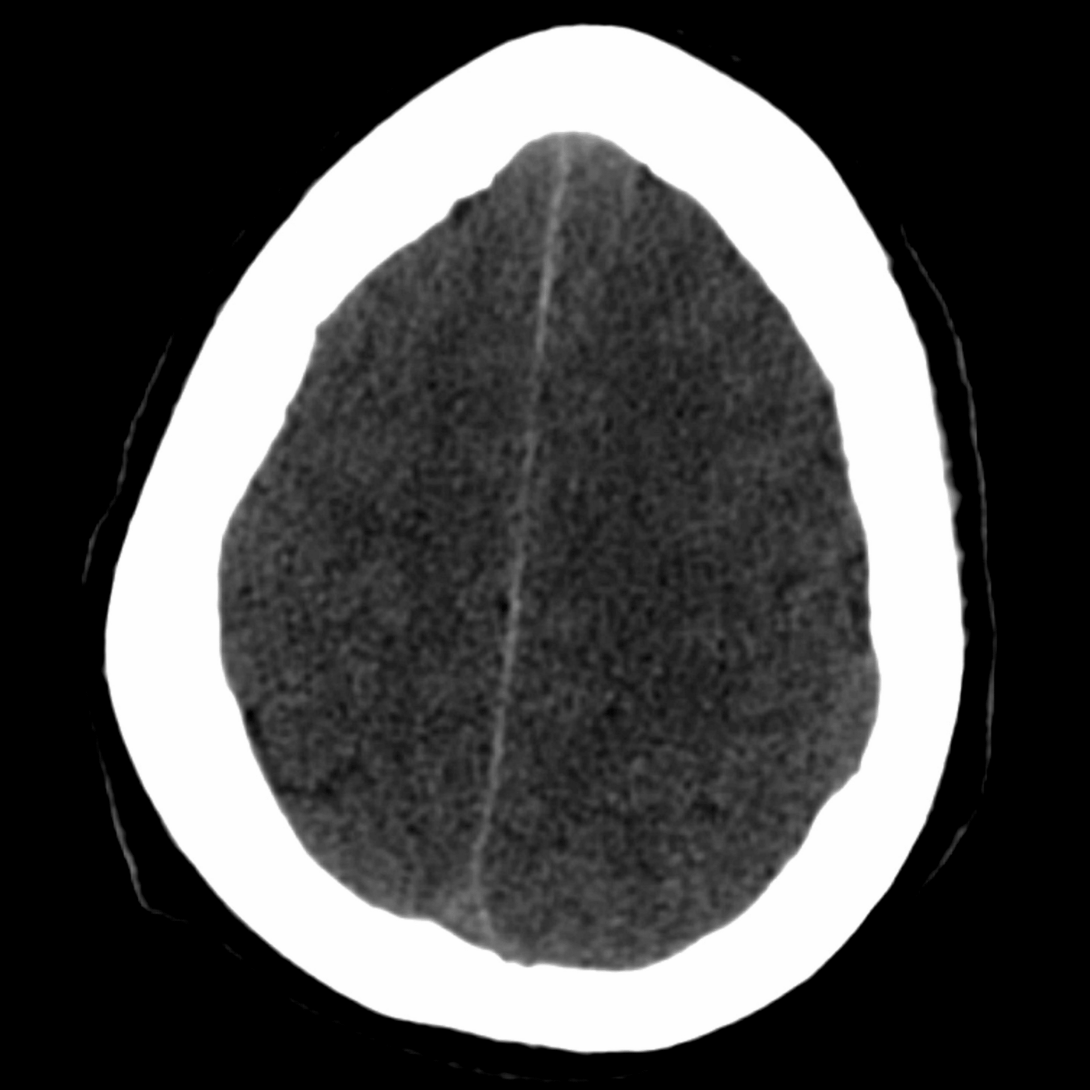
CT scan of the brain CT of the brain shows diffuse loss of CSF spaces along the cerebral convexities and blurring of the gray-white matter junction, indicating cerebral edema.
